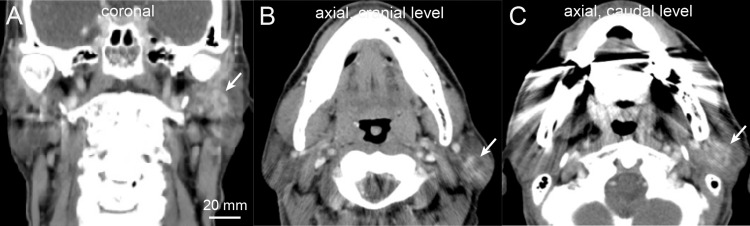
Computed tomography (CT) findings. (A) Coronal CT image. (B) Center: Axial CT image at the level of the cranial component. (C) Axial CT image at the level of the caudal component. Arrows indicate the left intraparotid tumor. The boundary between the cranial and caudal components is obscured by dental metallic artifacts.
